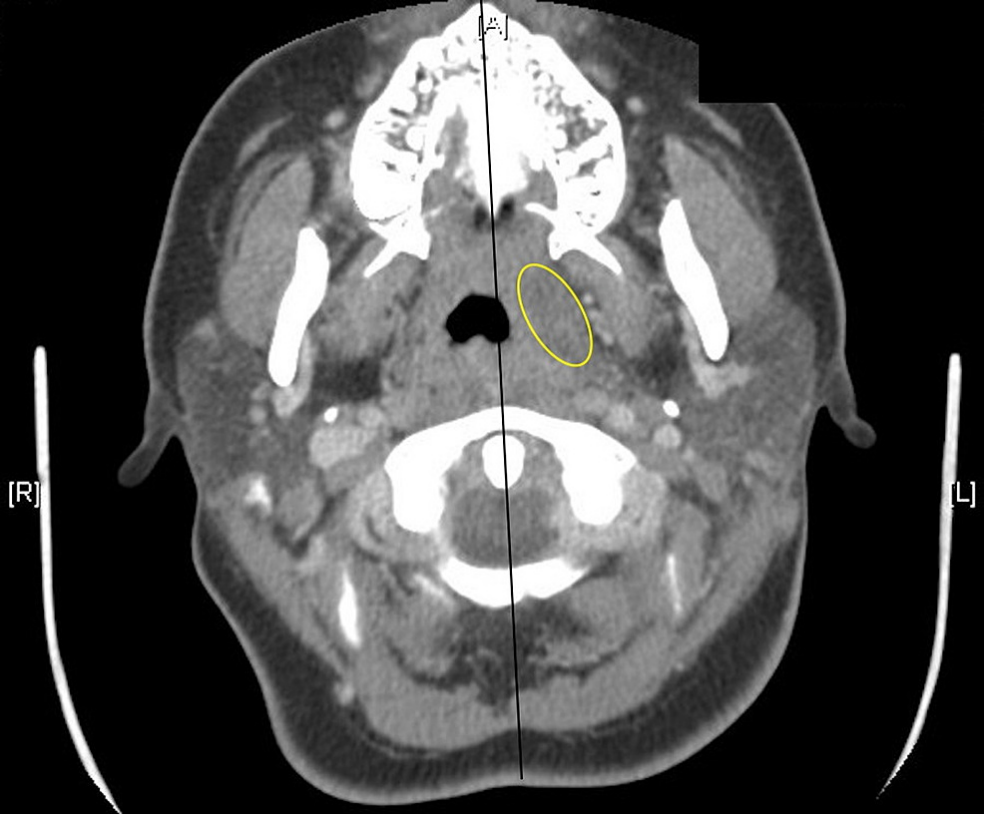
Axial-view computed tomography image demonstrating midline shift and tonsillar abscess on the left (yellow oval)
